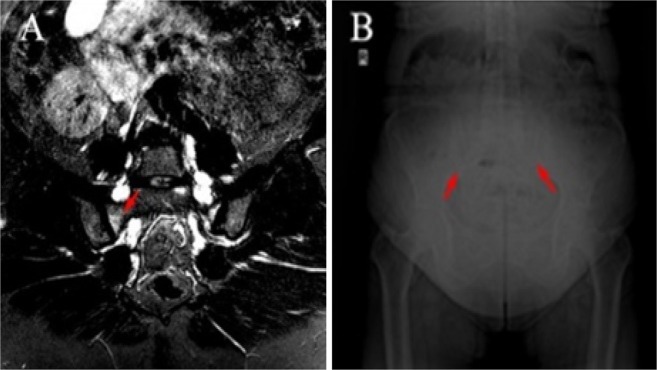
(A) STIR (short tau inversion recovery) sequence of MRI showing bone marrow edema in the sacroiliac joint of an acromegaly patient. (B) X-ray film showing sclerosis and partial ankylosis in the sacroiliac joint of an acromegaly patient.
